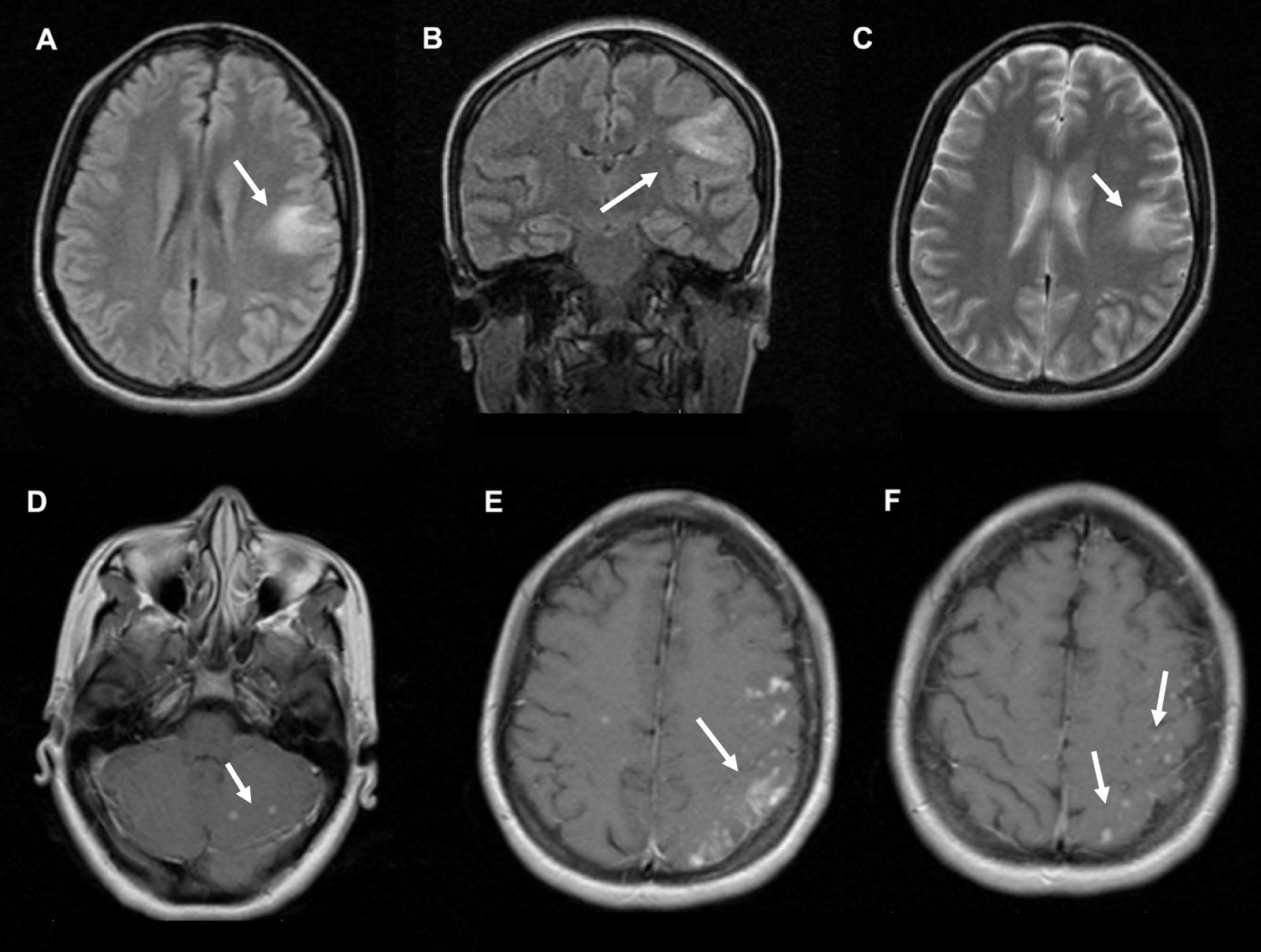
Case magnetic resonance image (MRI) (A) Axial MRI in FLAIR sequence showing a left temporal hyperintense lesion of the white matter in the left temporal lobe. (B) Coronal FLAIR sequence with inferior temporal hyperintense lesion of the white matter. (C) Axial T2-weighted sequence with left temporal hyperintensity. (D) Axial contrast-enhanced T1-weighted sequence showing nodular enhancing lesions on the cerebellum. (E) Axial contrast-enhanced T1-weighted sequence with cortical and nodular enhancing lesions in the left temporal lobe. (F) Axial contrast-enhanced T1-weighted sequence showing cortical nodular enhancing lesions. FLAIR: fluid-attenuated inversion recovery.
